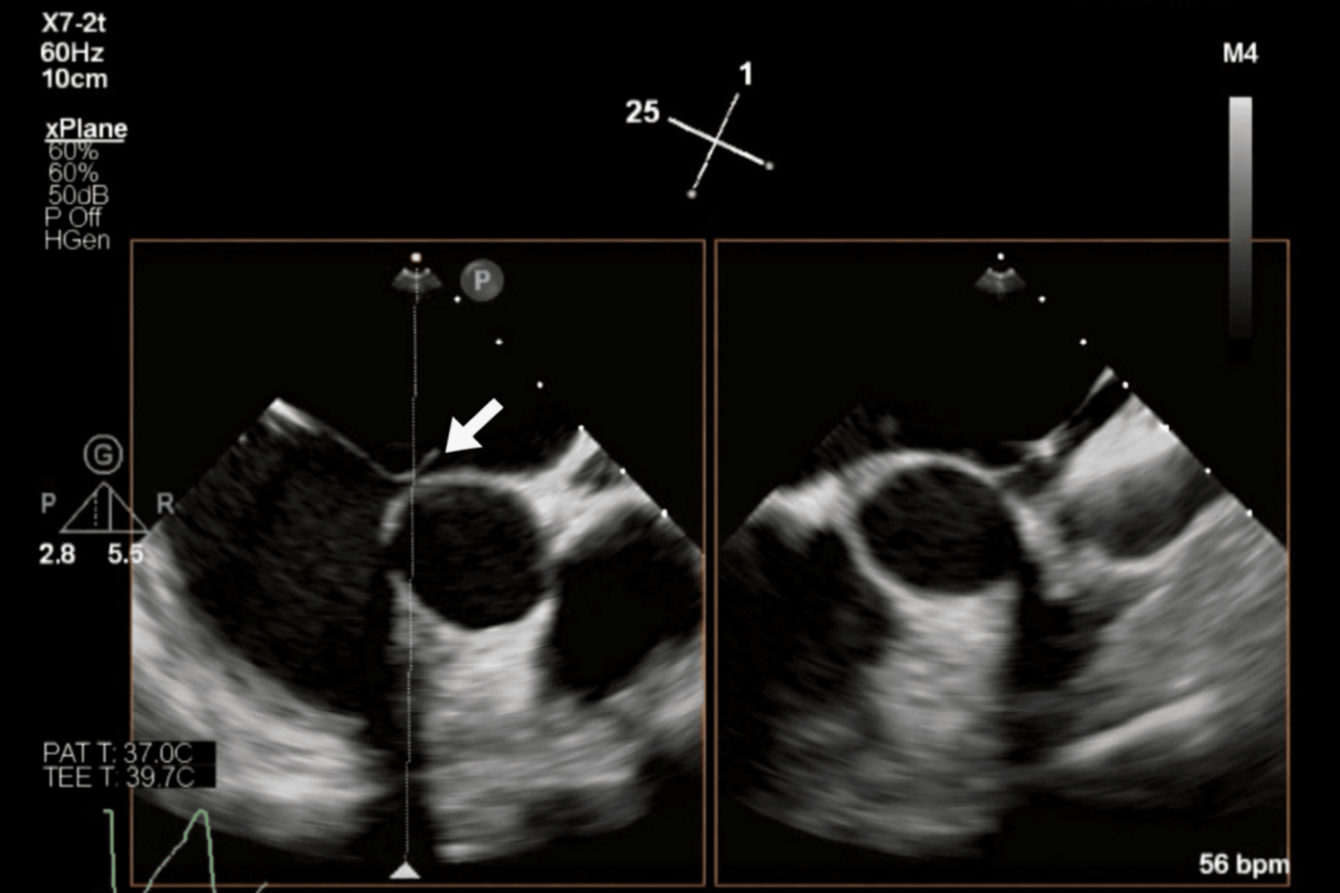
Transesophageal echocardiography performed during diagnostic workup Arrow indicated a large patent foramen ovale
